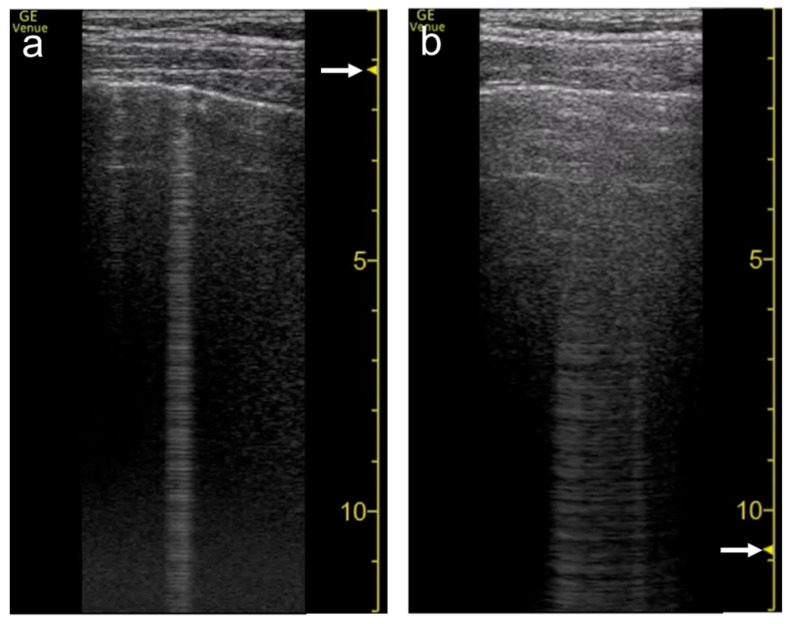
Ultrasound images obtained by Venue scanner (GE Healthcare) with a linear transducer. The B-line becomes wider as the single focal point (arrow) is shifted from the same level as the pleural line (a) to a deep level (b).
